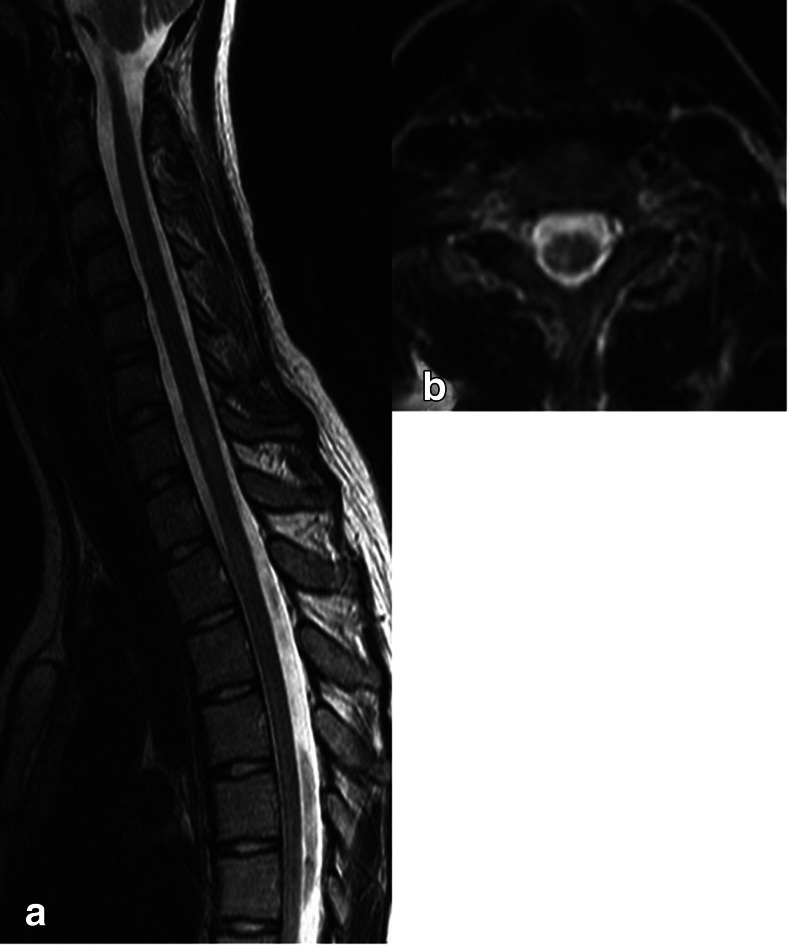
Case 2. a. Sagittal T2-weighted image of the spinal cord demonstrates two lesions located on C7, and Th2 levels. b. axial T2-weighted image shows hyperintense lesions located on the right side of the thoracic spinal cord
